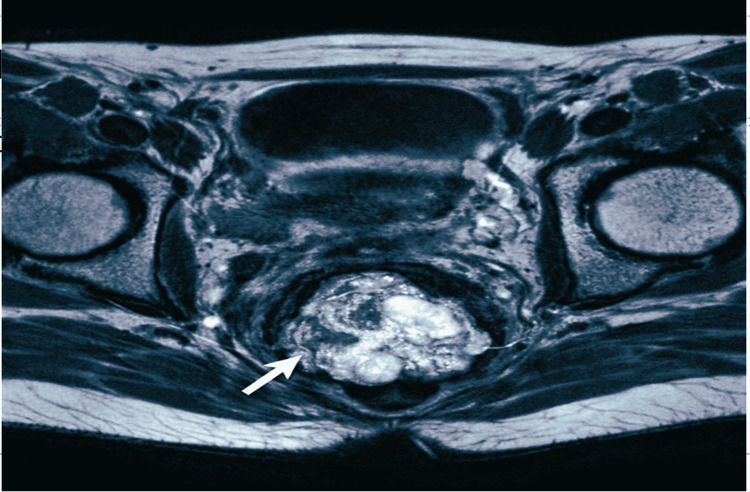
MRI pelvic axial T2-weighted image. MRI pelvic axial T2-weighted image demonstrating a lobulated vulvar lesion with heterogeneous signal intensity (arrow), measuring approximately 57 × 54 mm with a craniocaudal extension of about 45 mm. No extension to the vagina, urethra, or pelvic lymph nodes was observed, supporting the diagnosis of an isolated vulvar metastatic lesion. MRI: magnetic resonance imaging
